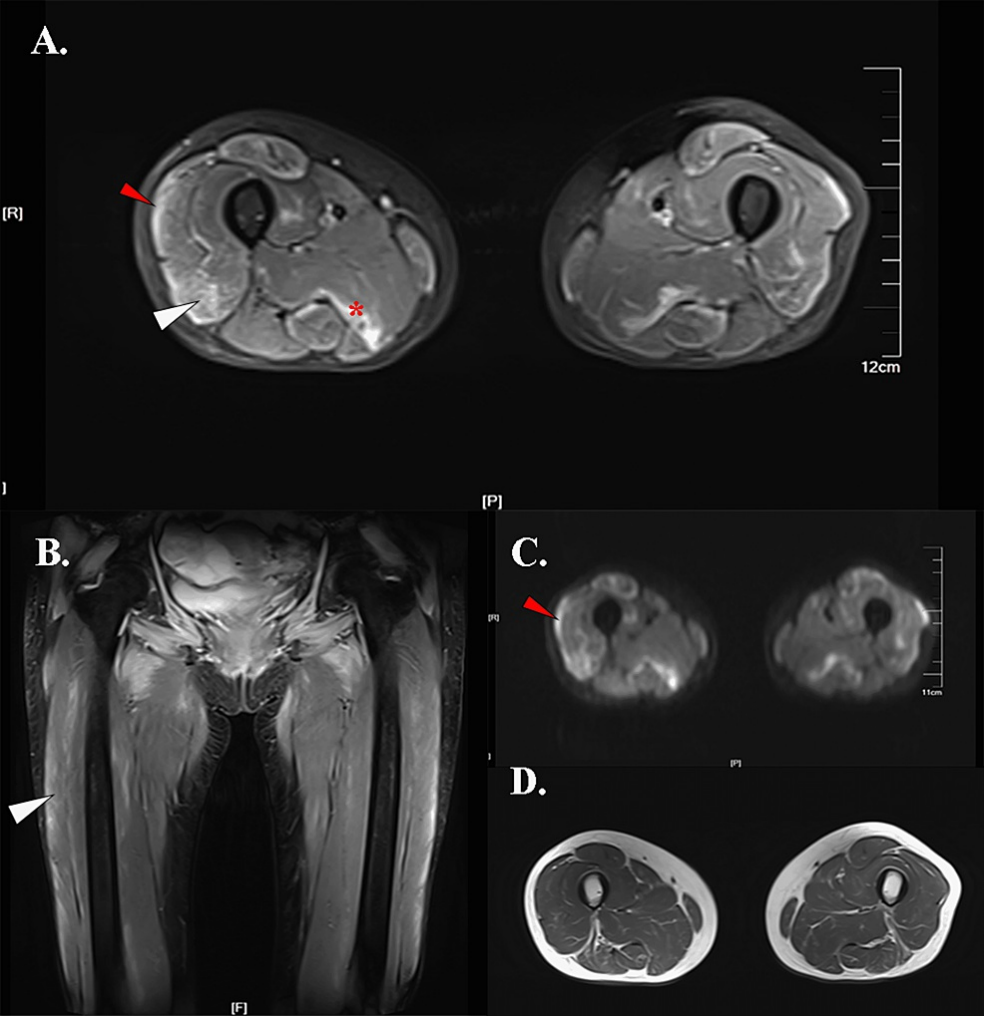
Thigh muscle MRI images of Case 2 A. A STIR sequence image showed fascia involvement (red arrow), peripheral honeycomb edema signal of vastus lateralis (white arrow), and peripheral foggy edema signal of the adductor magnus (red *). B. A coronal STIR sequence image showed a vastus lateralis edema signal (white arrow). C. An axial DWI sequence image showed a fascia hyperintensity signal (red arrow). D. An axial T1 sequence image showed no fatty infiltration. Abbreviations: STIR, short time of inversion recovery; DWI, diffusion-weighted imaging
